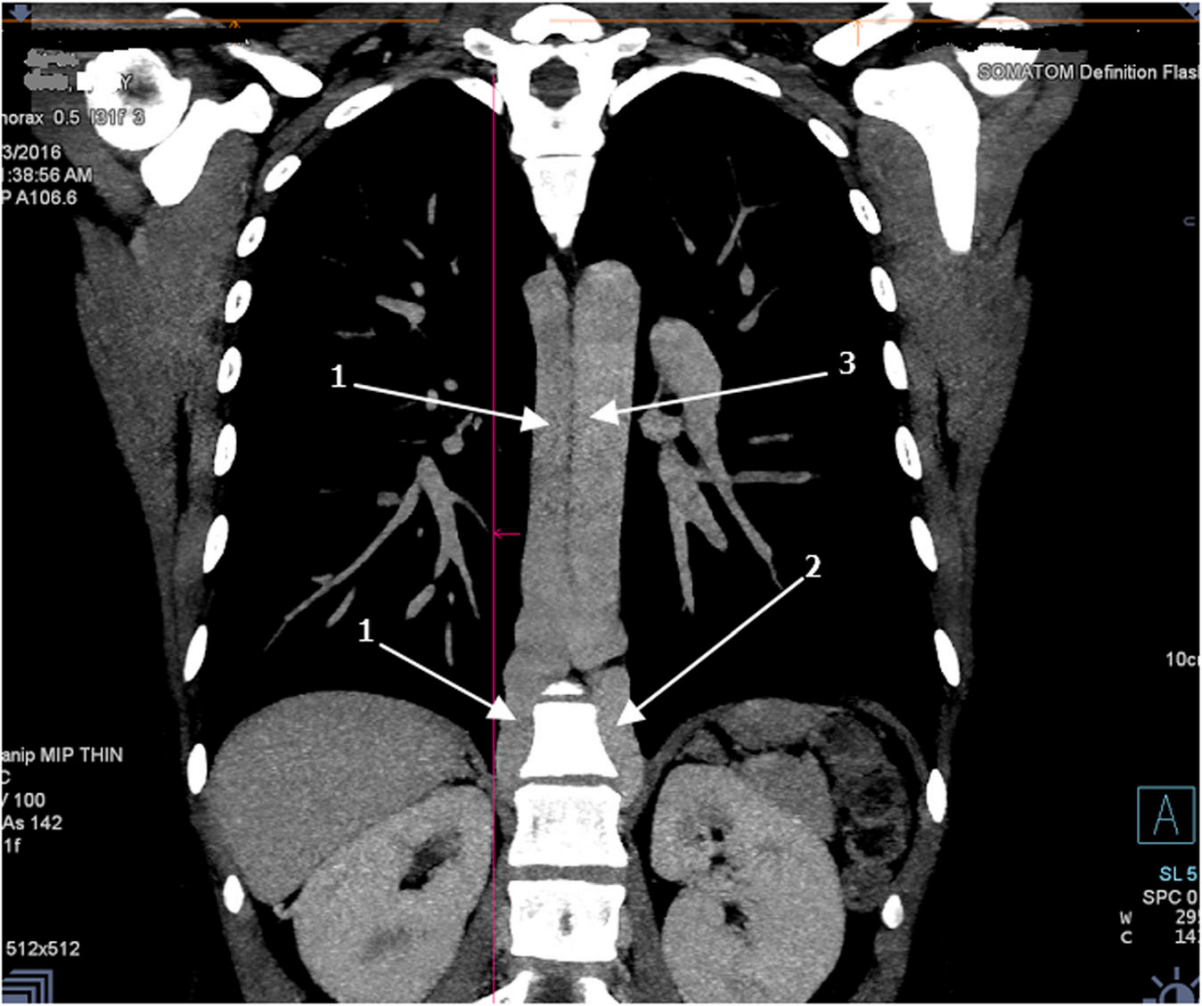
Computed tomography angiography (coronal view) of the thoracic region displaying tortuosity and dilatation of the azygos vein. *1* azygos vein, *2* hemiazygos vein, *3* aorta
